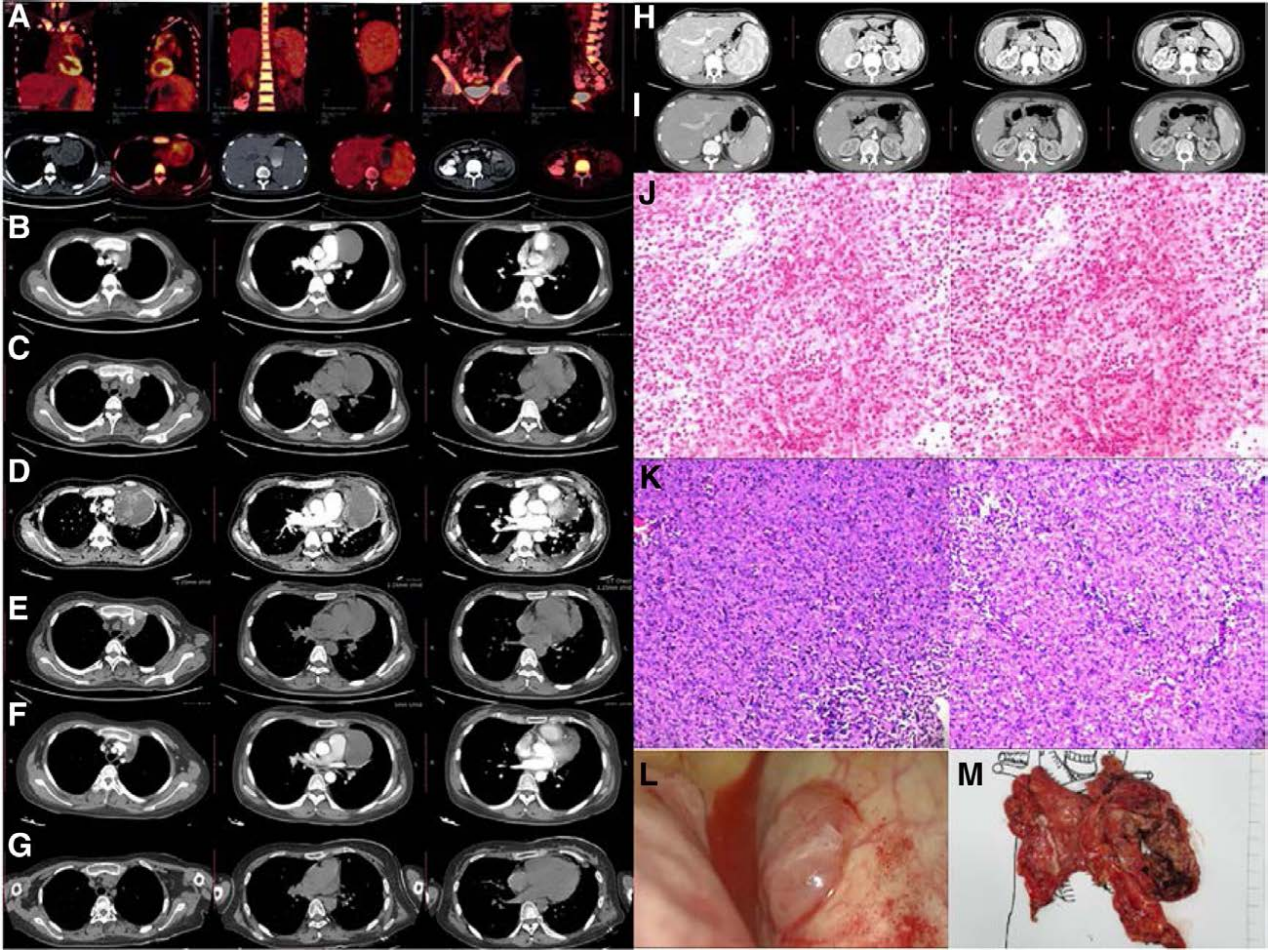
The PET/CT of the patient, huge anterior mediastinum mass, increased metabolism, secondary pericardial effusion and left pleural effusion, enlarged lymph nodes in the hepatic portal and retroperitoneum with increased metabolism, enlarged spleen with active metabolism (A). Evolution of the chest CT of the patient during the Tianjin Medical University General Hospital, September 29, 2020, the chest CT revealed that the anterior mediastinal mass was a multilocular cystic solid mass with a maximum cross-sectional area of about 6.5 × 7.0 cm (B). October 27, 2020, the chest CT revealed that the anterior mediastinal multilocular cystic solid mass was slightly larger than the previous one, and the largest cross-sectional area was about 6.6 × 7.2 cm (C). January 5, 2021, the chest CT revealed that the multilocular cystic solid mass was significantly larger than before, with a maximum cross-sectional area of about 13.1 × 8.1 cm, with clear boundaries. The mass surrounded the main pulmonary artery and locally protruded into the upper left lung leaf (D). January 22, 2021, the chest CT revealed that the multilocular cystic solid mass was smaller than before, and the size of the patient’s anterior mediastinum mass was about 8.1 × 6.0 cm (E). February 19, 2021, the chest CT revealed that the maximum cross-sectional area was about 8.1 × 6.0 cm (F). March 9, 2021, the chest CT revealed that the patient’s chest condition after surgery (G). Evolution of the CT of abdomina the patient during the Tianjin Medical University General Hospital on January 5, 2021 (H) and February 19, 2021 (I). Thymoma biopsy (J, K). During the operation, several nodules were found in the chest wall and diaphragm muscle (L) and resected tumor (M). CT = computed tomography, PET/CT = positron emission tomography/ computed tomography.
